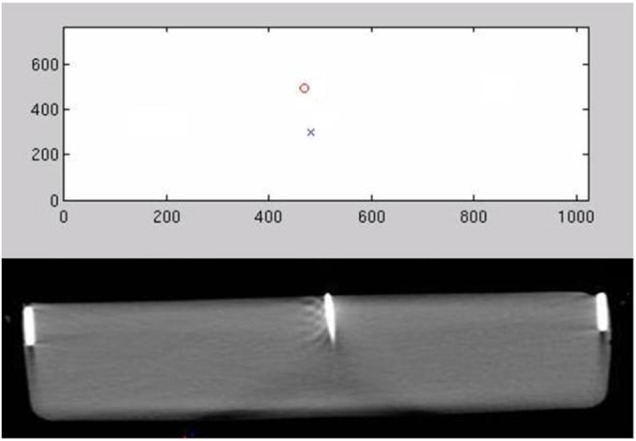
Location of the needle tip identified using two infrared cameras, with the true DICOM CT-scanned image below. In the top image, the x and o are live outputs from the IR cameras, which demonstrate the location of the needle using the reflection from two infrared reflective tapes. The dimensions of the top image are the raw locations from the IR camera, which are converted to an image location using the method proposed in this paper
